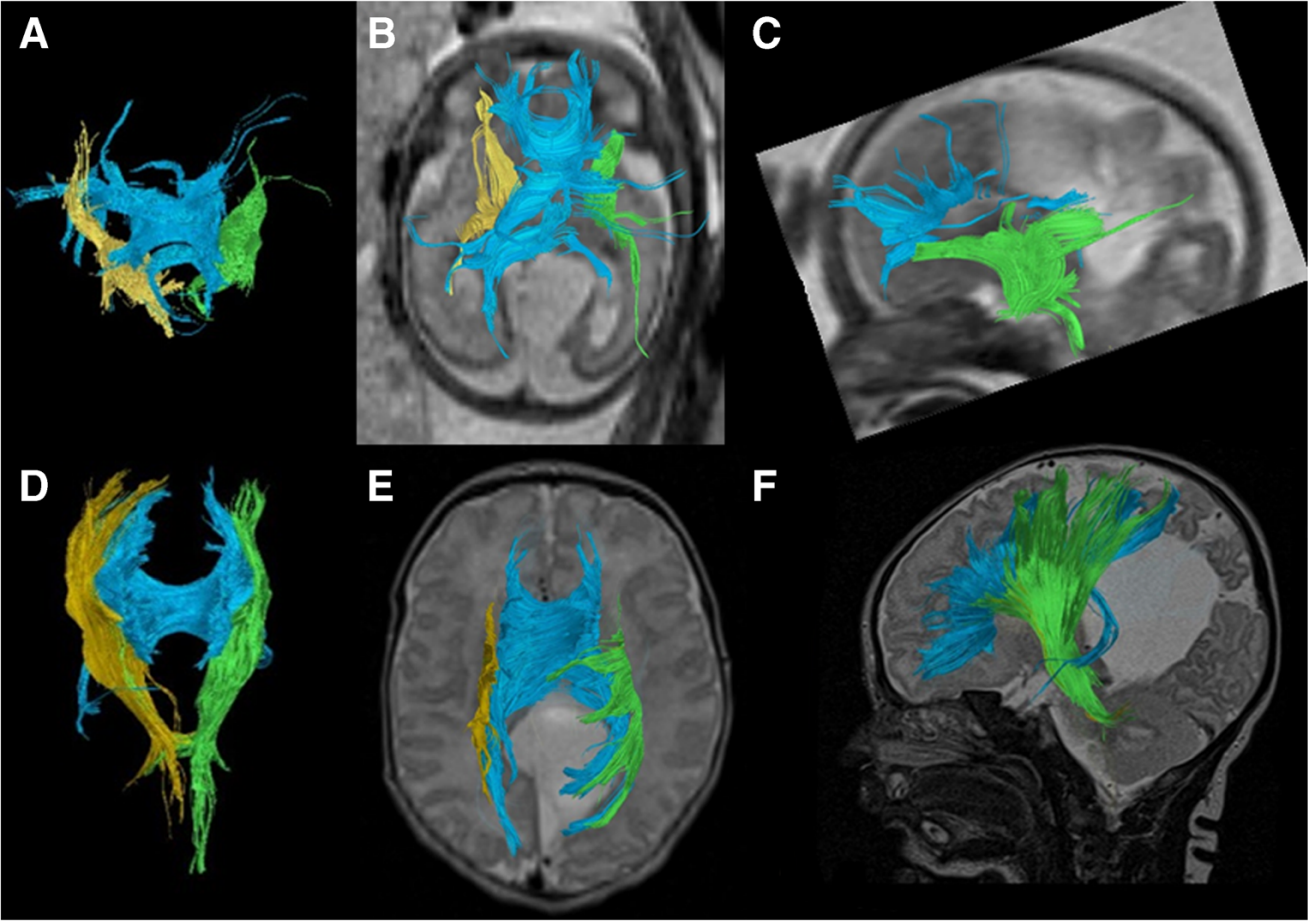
Fetal and postnatal corpus callosum and corticospinal tract segmentation in a boy (case 9) with a midline parieto-occipital cyst. a–c Age 29+4 gestational weeks. a Coronal diffusion tensor imaging (DTI) of segmented right (*yellow*) and left (*green*) corticospinal tracts superimposed with the corpus callosum (*blue*) in the fetal brain. b Overlay of the segmented fiber tracts on an axial T2-weighted image. c Sagittal view of the left corticospinal tract (*green*) reveals a tract that appears intact. Partially viewed is the corpus callosum (*blue*) as well on this T2-weighted image of the fetal brain. d–f Age 5 days. d Coronal DTI image of segmented right (*yellow*) and left (*green*) corticospinal tracts superimposed with the corpus callosum (*blue*) in the postnatal brain. e Axial view of the segmented tracts on a T2-weighted image shows lateral displacement of the fiber tracts posteriorly from the midline cyst. f Overlay of the segmented left corticospinal (*green*) and corpus callosum (*blue*) tracts on a sagittal T2-weighted image of the postnatal brain
